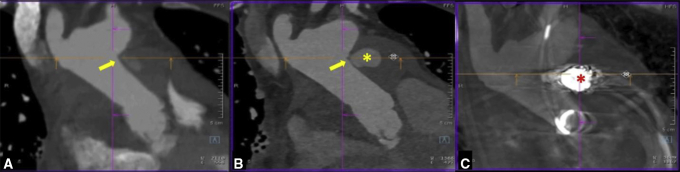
Embolization of the ascending aortic pseudoaneurysm. A, Initial cardiac computed tomography angiography on re-admission demonstrated a 4-cm ascending aortic pseudoaneurysm (*yellow arrow*). B, Repeat imaging after attempted endovascular embolization demonstrated interval increase in size with more contrast extravasation (*yellow asterisk*) in the periaortic region. C, Following percutaneous embolization under image fusion guidance, cone-beam computed tomography with contrast injection showed no extravasation or residual contrast filling of the pseudoaneurysm, and a coil mass (*red asterisk*) overlapping the region of original contrast extravasation.
